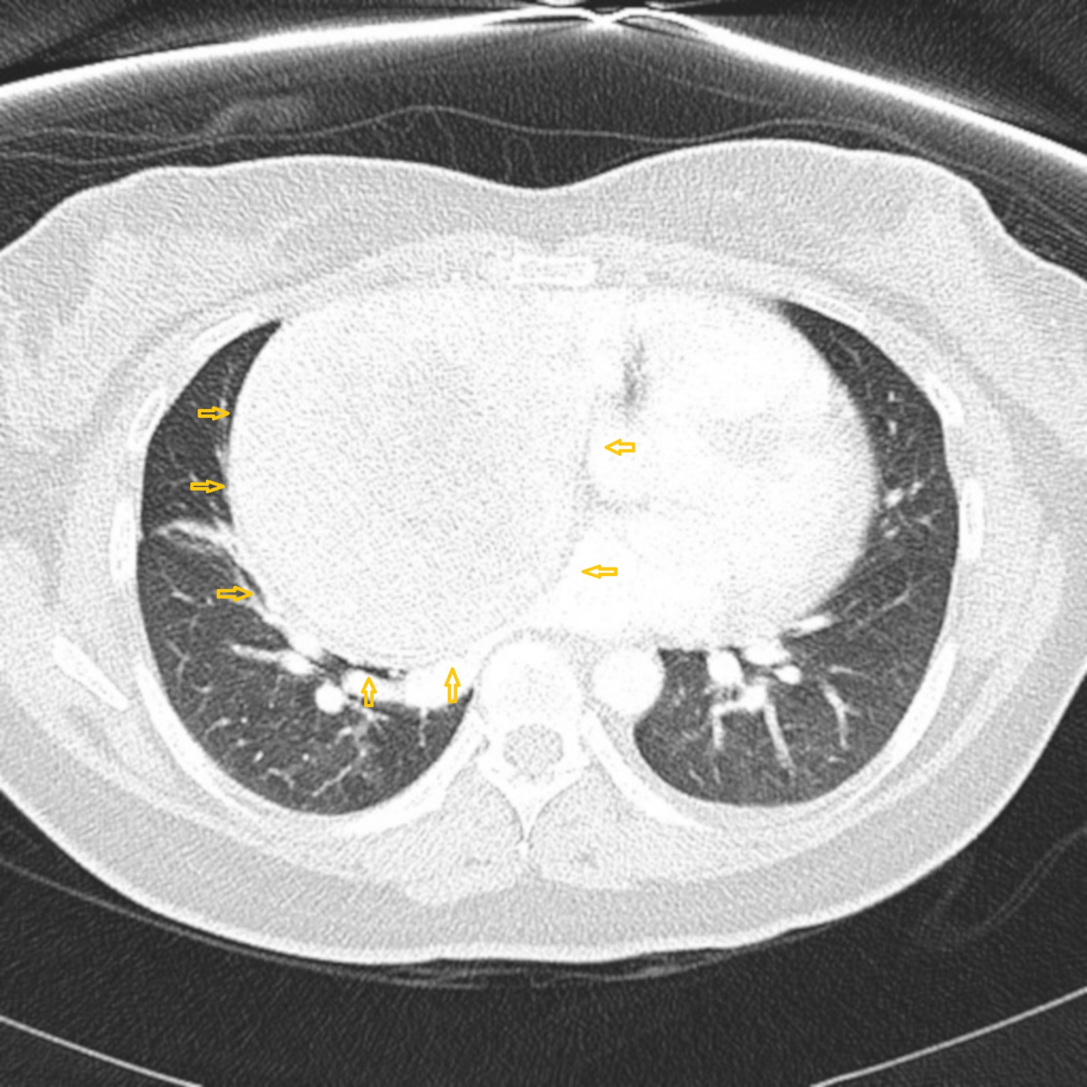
CT chest with contrast showing a large, well-circumscribed, heterogeneously enhancing mass showing peripheral calcification in the right mediastinum CT: computed tomography
